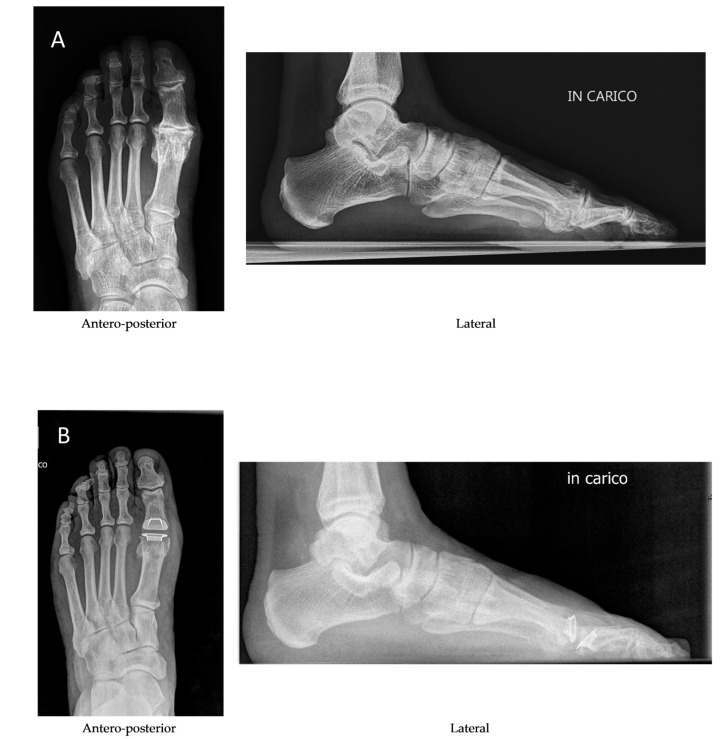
(A) Preoperative and (B) postoperative X-ray weight bearing.
